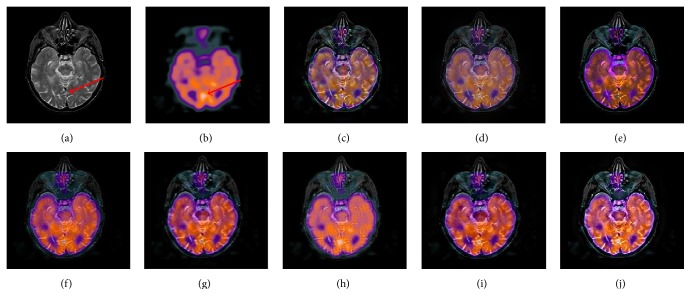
The fusion results of different fusion methods for the second set of MRI and SPECT images: (a), (b) Source images; fused images by (c) DWT, (d) GP, (e) IHS, (f) ST, (g) CT, (h) NSST-4, (i) NSCT, and (j) the proposed method.
